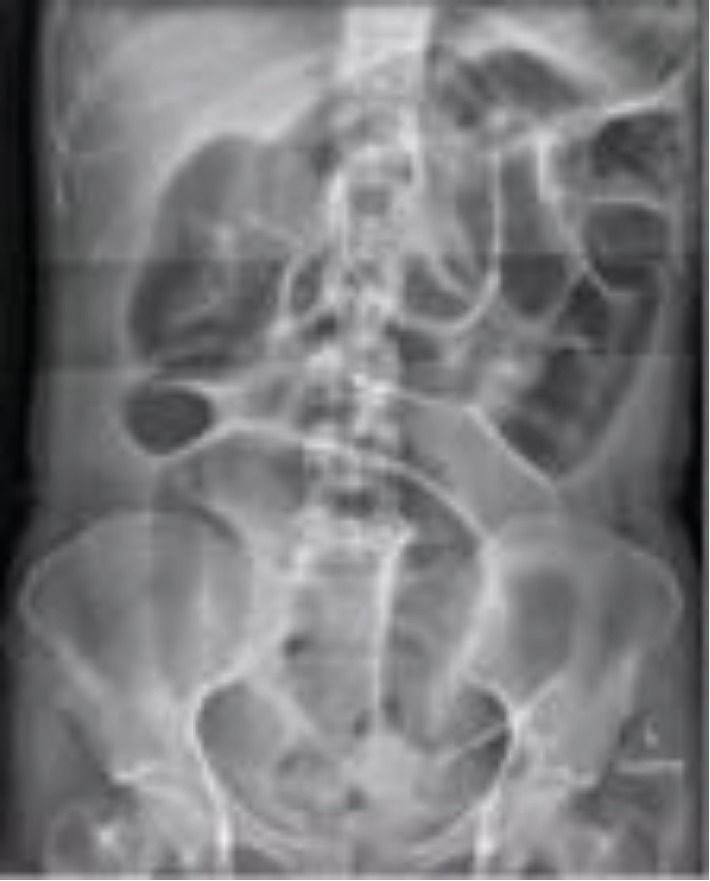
Abdominal X-ray image demonstrating multiple dilated loops of the small bowel.
